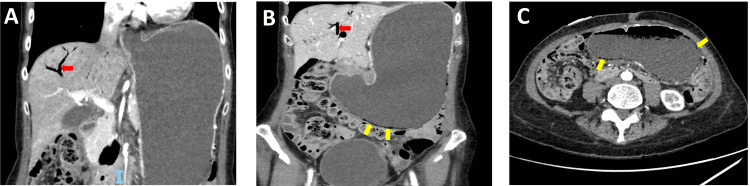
Coronal CT imaging demonstrating a grossly distended stomach with air in the portal venous system (red arrows in A and B); cross-sectional CT imaging showing air in the gastric wall (yellow arrows in B and C)
